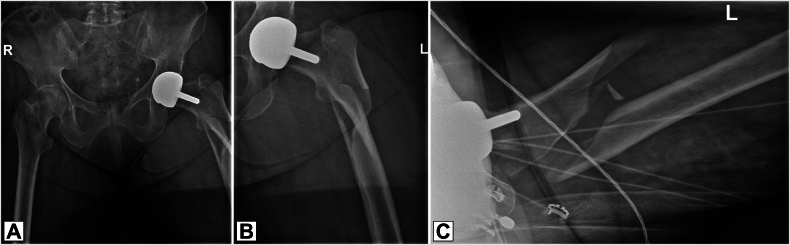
Preoperative radiographs, showing a left subtrochanteric femoral fracture with slight dislocation, distal to a Birmingham Hip Resurfacing prosthesis. A: Anterior-Posterior (AP) X-ray of pelvis. B: AP X-ray of left femur. C: Axial X-ray of left femur.
